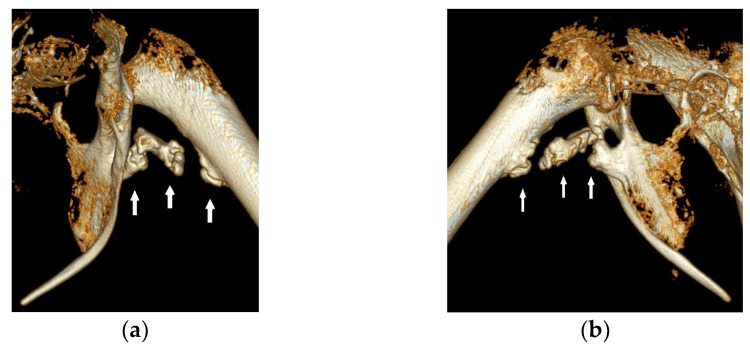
Cranial (a) and caudal (b) view of 3D computed tomographic reconstruction of the heterotopic bone formations (arrows) of the adductors of the bald eagle of case 1.
